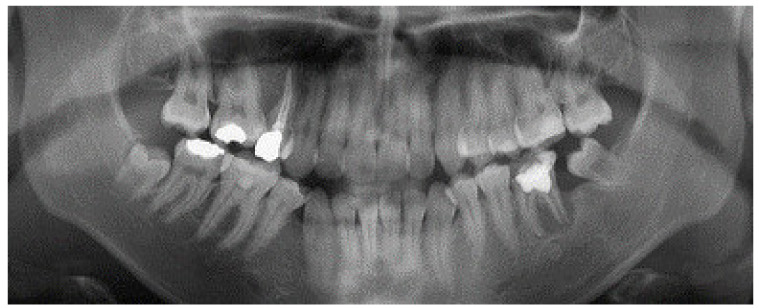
The radiographic evaluation showed severe bone loss in the distal root area of the hopeless tooth #19.
